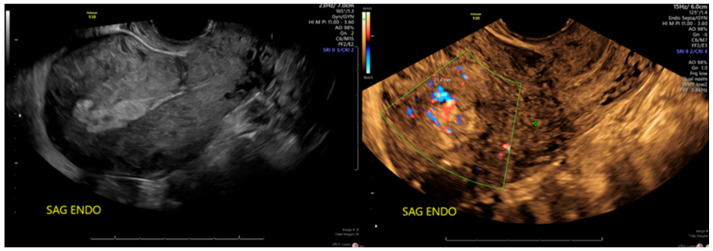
Ultrasound obtained 2 weeks after hysteroscopy and dilatation and curettage, showing persistent 2.5 × 2.0 cm area of thickened tissue with irregular borders and areas of vascularity suggesting focal placenta accreta.
